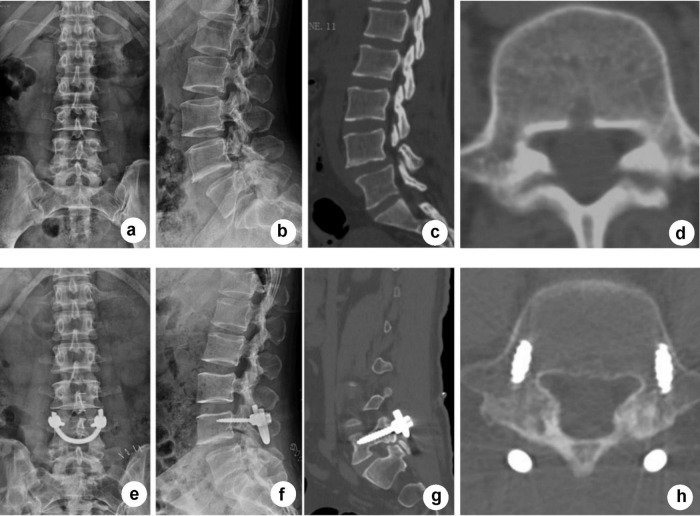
Typical case of SFR. A 37-year-old male patient who had suffered from low back pain for 4 years underwent SFR surgery. The pain was significantly reduced 1 year after surgery. (a,b) Anteroposterior and lateral X-ray CT before surgery showed L5 spondylolysis with grade I spondylolisthesis. (c,d) Sagittal and axial CT images before surgery showed L5 bilateral spondylolysis. (e,f) Anteroposterior and lateral X-ray after surgery showed that SFR provided strong fixation on the lesion. (g,h) CT showed that L5 spondylolysis reached preliminary fusion 1 year after surgery.
